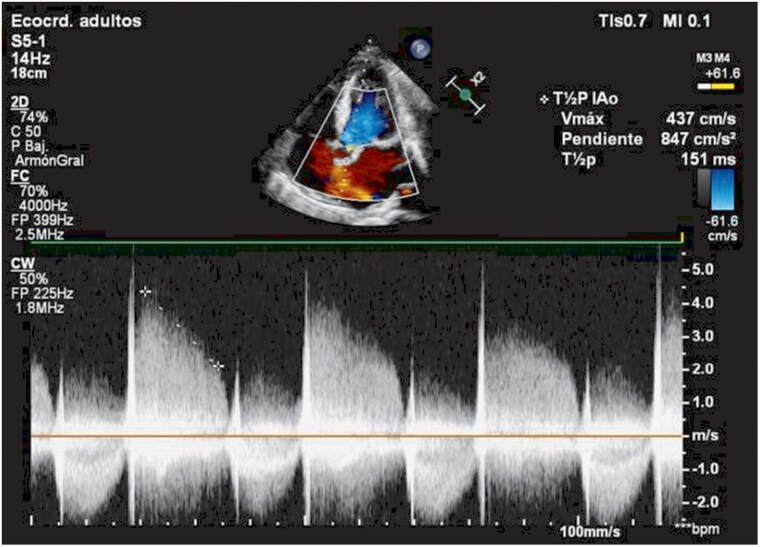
Transthoracic echocardiography with colour Doppler study showing multiple eccentric aortic regurgitation jets directed towards the anterior mitral leaflet, reaching the apex of the left ventricle. Pressure half-time was 151 ms.
